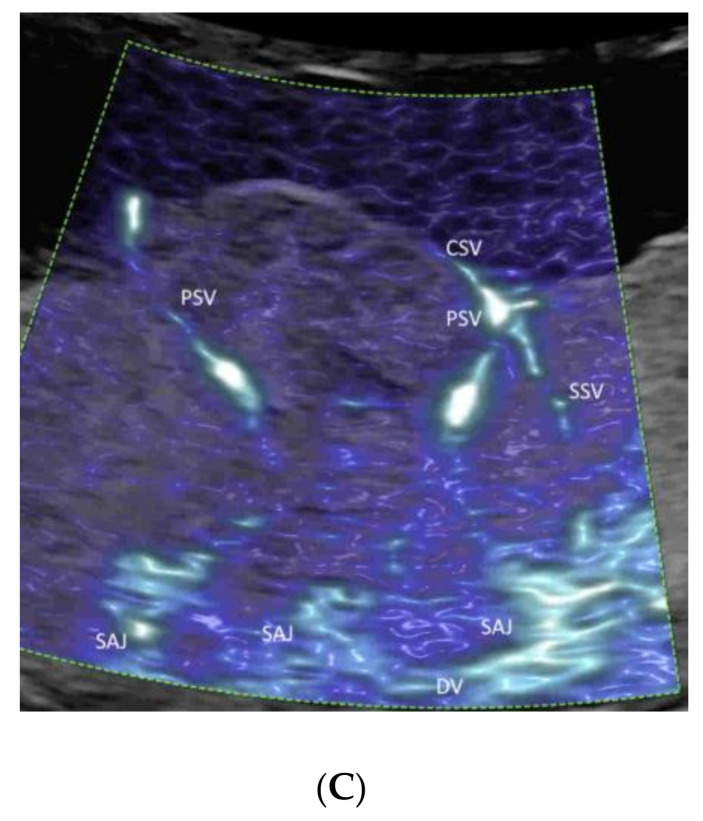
Color Doppler (A), high-definition (B) and microvascular (C) flow imaging of placental vessels in a normal pregnancy at 23 weeks’ gestation. Note the demonstration of the spiral artery jet (SAJ), as well as the primary (PSV) and secondary (SSV) stem villi in (C). DV, decidual vessels, CSV, chorionic surface vessels; AF, amniotic fluid.
